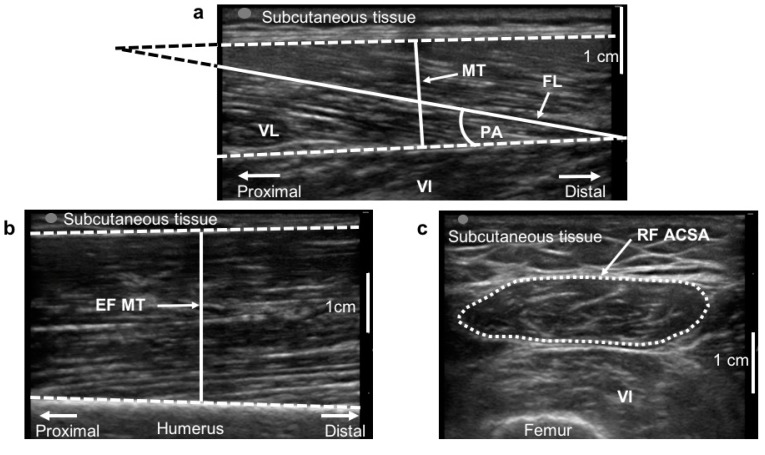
Ultrasound images analysis. Ultrasound images analysis for vastus lateralis (VL, a), elbow flexors (EF, b) and rectus femoris (RF, c) muscles. (a): muscle thickness (MT), fascicle length (FL) and pennation angle (PA) are highlighted. The non-visible part of FL was extrapolated as shown. (b): elbow flexors MT. (c): the anatomical cross-sectional area (ACSA) of RF is represented with a dotted line. VI, vastus intermedius.
